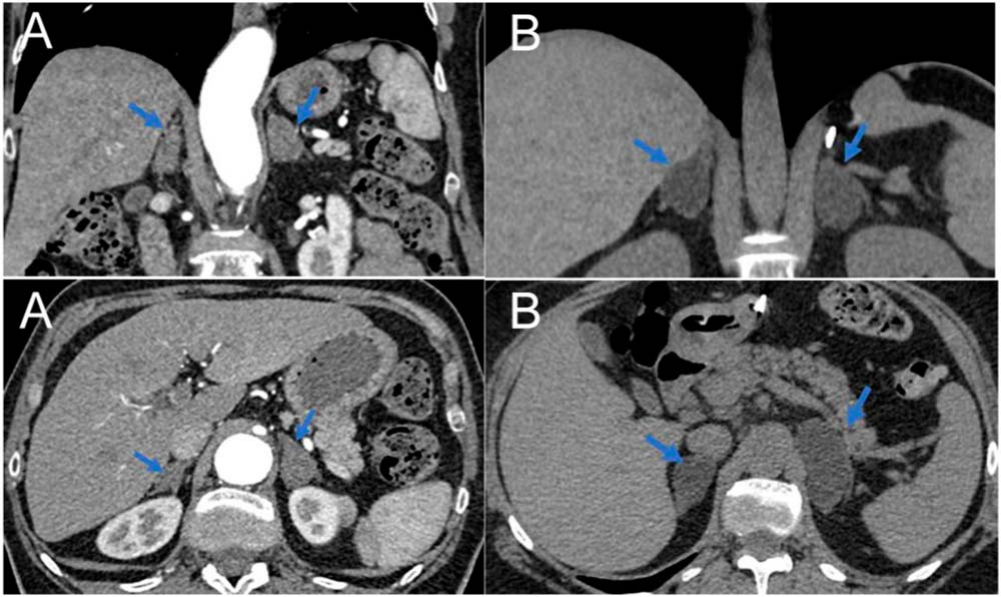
Abdominal CT scan with IV contrast. Coronal and axial images of the abdomen showing PBMAH with a right adrenal nodule of 35 × 15 mm and a left adrenal nodule of 20 × 27 mm for case 1 (A) and PBMAH with a right adrenal nodule of 22 × 28 mm and a left adrenal nodule of 24 × 50 mm for case 2 (B).
